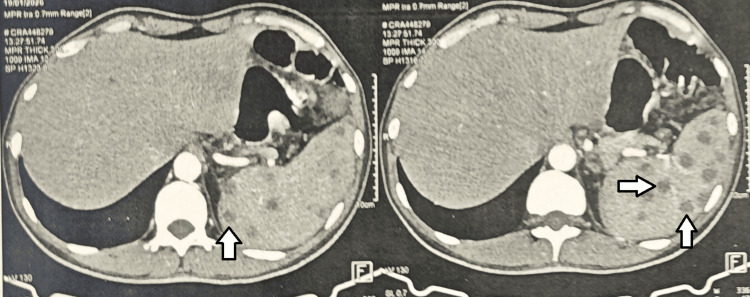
Axial abdominal CT demonstrates a normal-sized spleen containing multiple hypodense nodules measuring approximately 5-14 mm. The arrows highlight the focal splenic lesions corresponding to tuberculous involvement, helping to emphasize the abnormal areas of interest described in the radiological findings.
